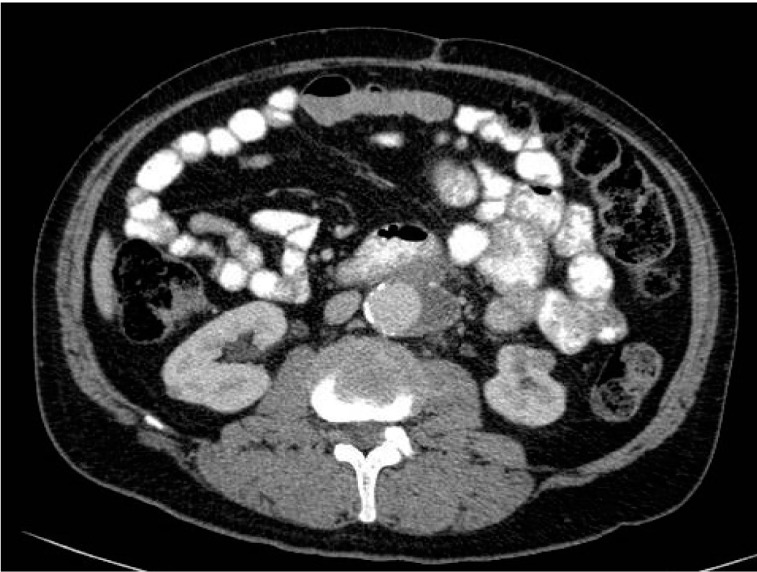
Computed tomography of renal tumour at three months after surgery with the vascular graft in situ and the lesion in lower pole of left kidney, showing cystic change and reduction in size (as a result of tumour necrosis following ligation of accessory renal artery)
